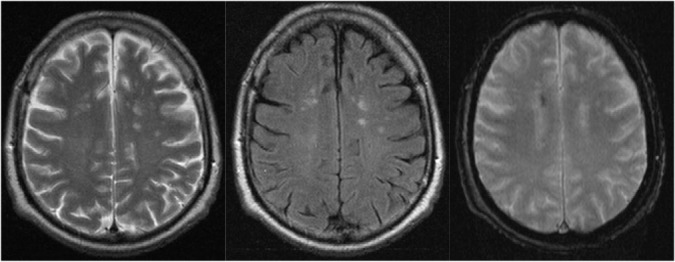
Brain MRI 8 months after treatment.
